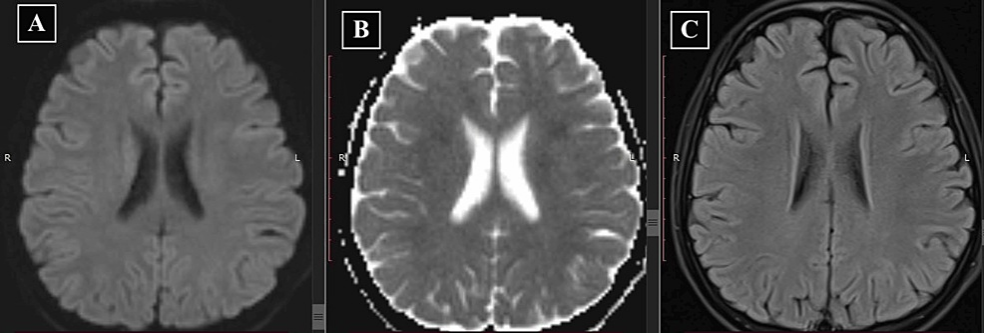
Follow-up MRI scan after one month (A) Normal diffusion-weighted MRI image. (B) Normal ADC map. (c) Normal FLAIR image. MRI, magnetic resonance imaging
